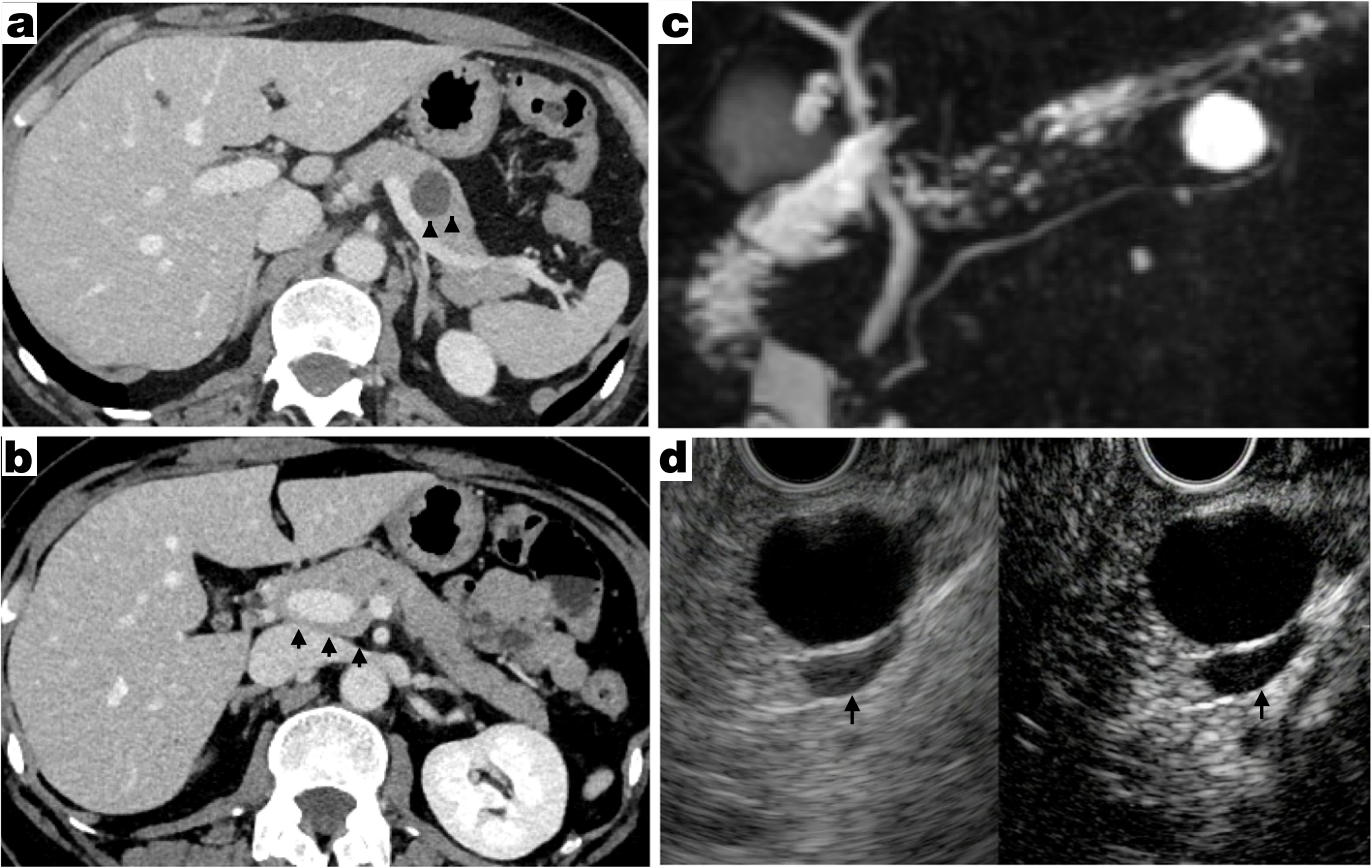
Preoperative imaging studies. Dynamic computed tomography scan showed oval cystic lesion of 1.5 cm in diameter of the pancreatic tail (a: arrow head) and portal annular pancreas (b: arrow). c Magnetic resonance cholangiopancreatography demonstrated the anteportal main pancreatic duct type. d Contrast-enhanced endoscopic ultrasound revealed oval cystic lesion without enhancement
